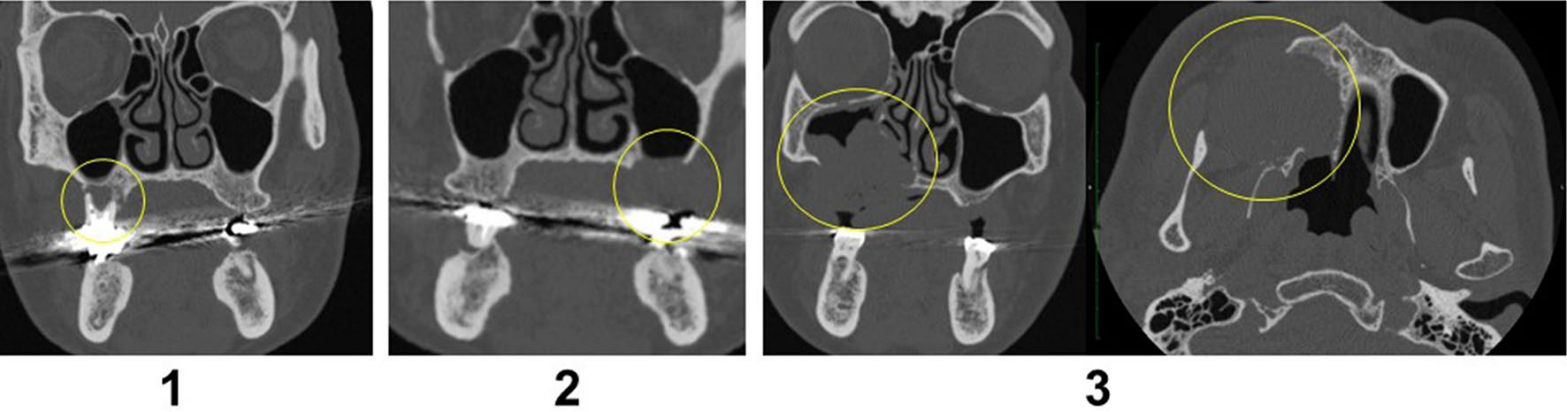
Bone Invasion Criteria. 0: no bone invasion, 1: invasion into maxilla, 2: invasion into sinus, 3: invasion into posterior maxillary wall. Bone invasion was diagnosed on axial, coronal, and sagittal CT images
